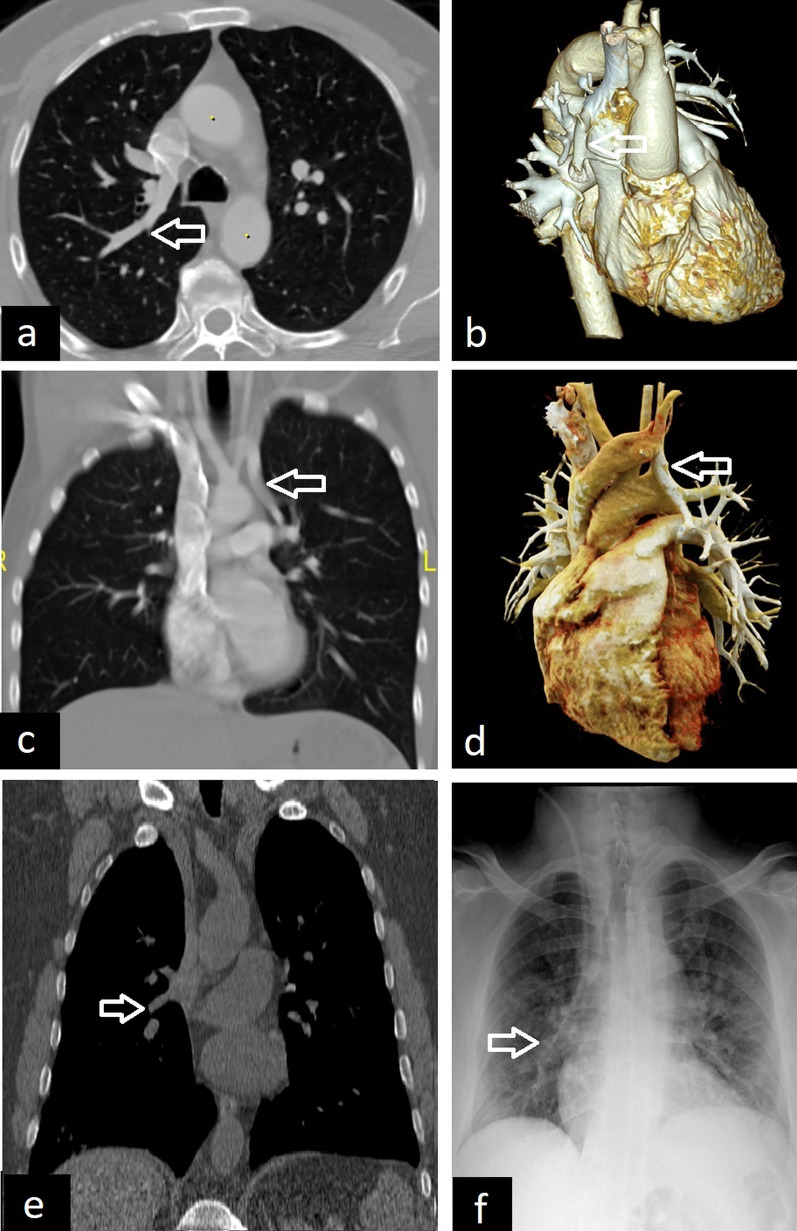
Different examples of partial anomalous pulmonary venous return (PAPVR). Axial CT (a) and 3D volume-rendered (b) images demonstrate anomalous right upper lobe pulmonary veins draining into the superior vena cava (arrows). The anomalous left upper lobe pulmonary vein draining into the brachiocephalic vein is observed on coronal CT (c) and 3D volume-rendered (d) images (arrows). An anomalous pulmonary vein is seen on coronal CT image (e, arrow) and central venous catheter malposition with the catheter tip extending towards the right lung in the same patient observed on chest X-ray (f, arrow)
